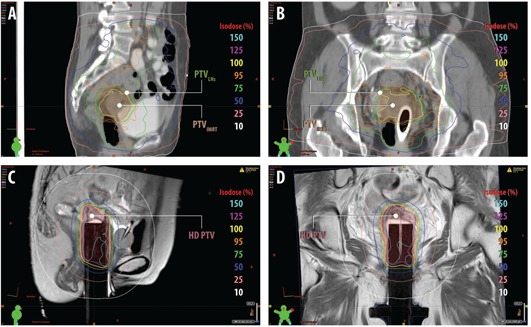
Isodose distributions on the (a) parasagittal and (b) paracoronal CT view for the approved external beam radiotherapy, and (c) parasagittal and (d) paracoronal T2‐weighted MR view for the approved HDR brachytherapy treatment plan. The external beam ${\text{PTV}}_{\text{IMRT}}$ and ${\text{PTV}}_{\text{LNs}}$ volumes are shown in the shadowed red and green contours, and the HDR PTV volume is shown in the shadowed red contour.
